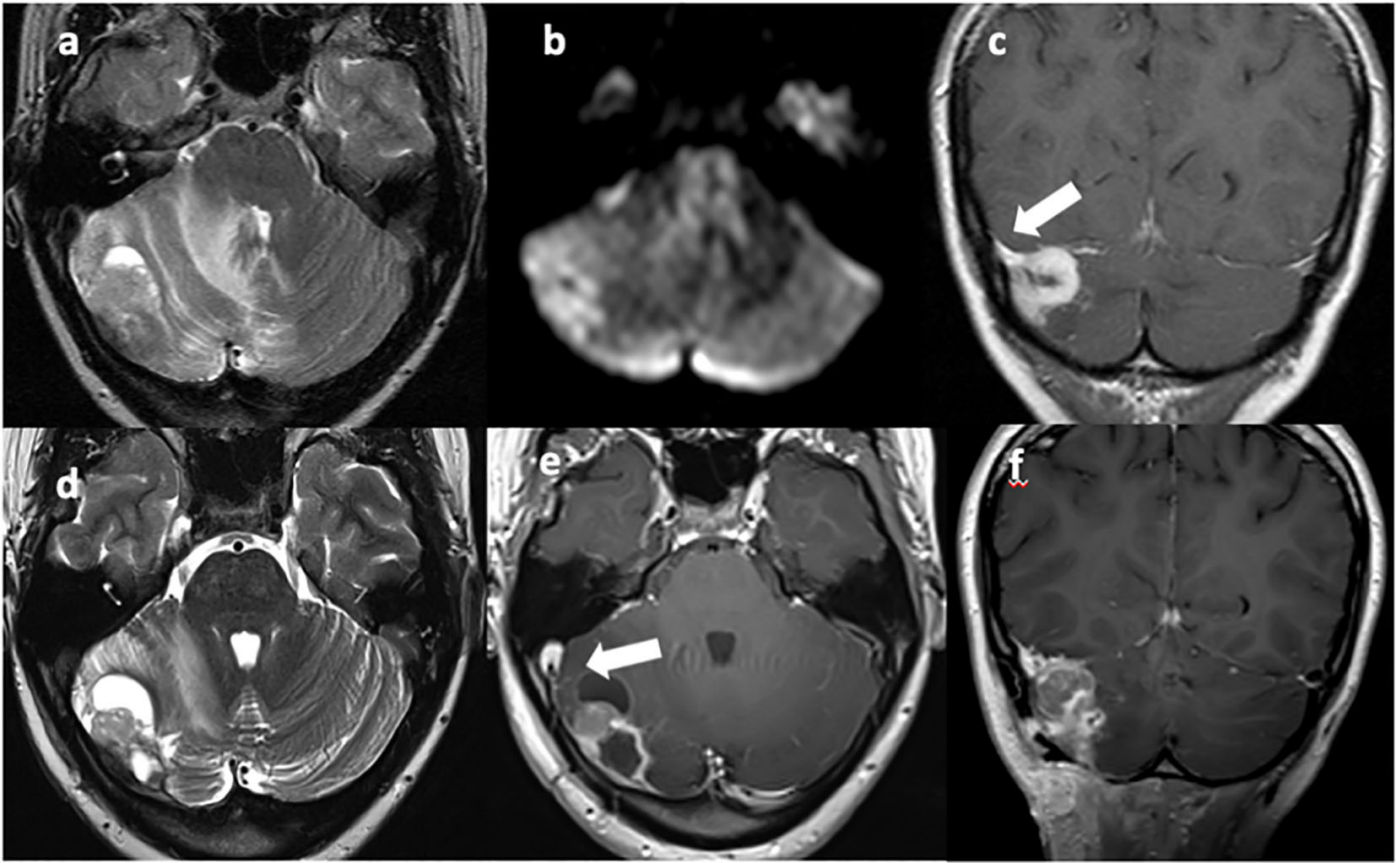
T2w axial (A, D) and T1w axial (E) and coronal (C, F) MRI images show a vascularized solid-cystic edematous lesion in the right cerebellar hemisphere, in continuity with the right transverse sinus [(C), arrow]. Diffusion restriction is present due to the high cellularity of the neoplastic tissue (B). Sinus thrombosis is also present [(E), arrow].
